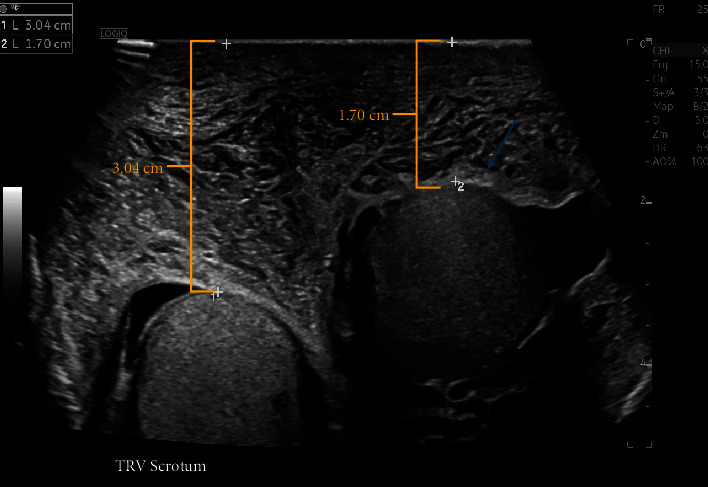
Scrotal ultrasound showing moderate to severe scrotal wall thickening consistent with diffuse scrotal edema.
